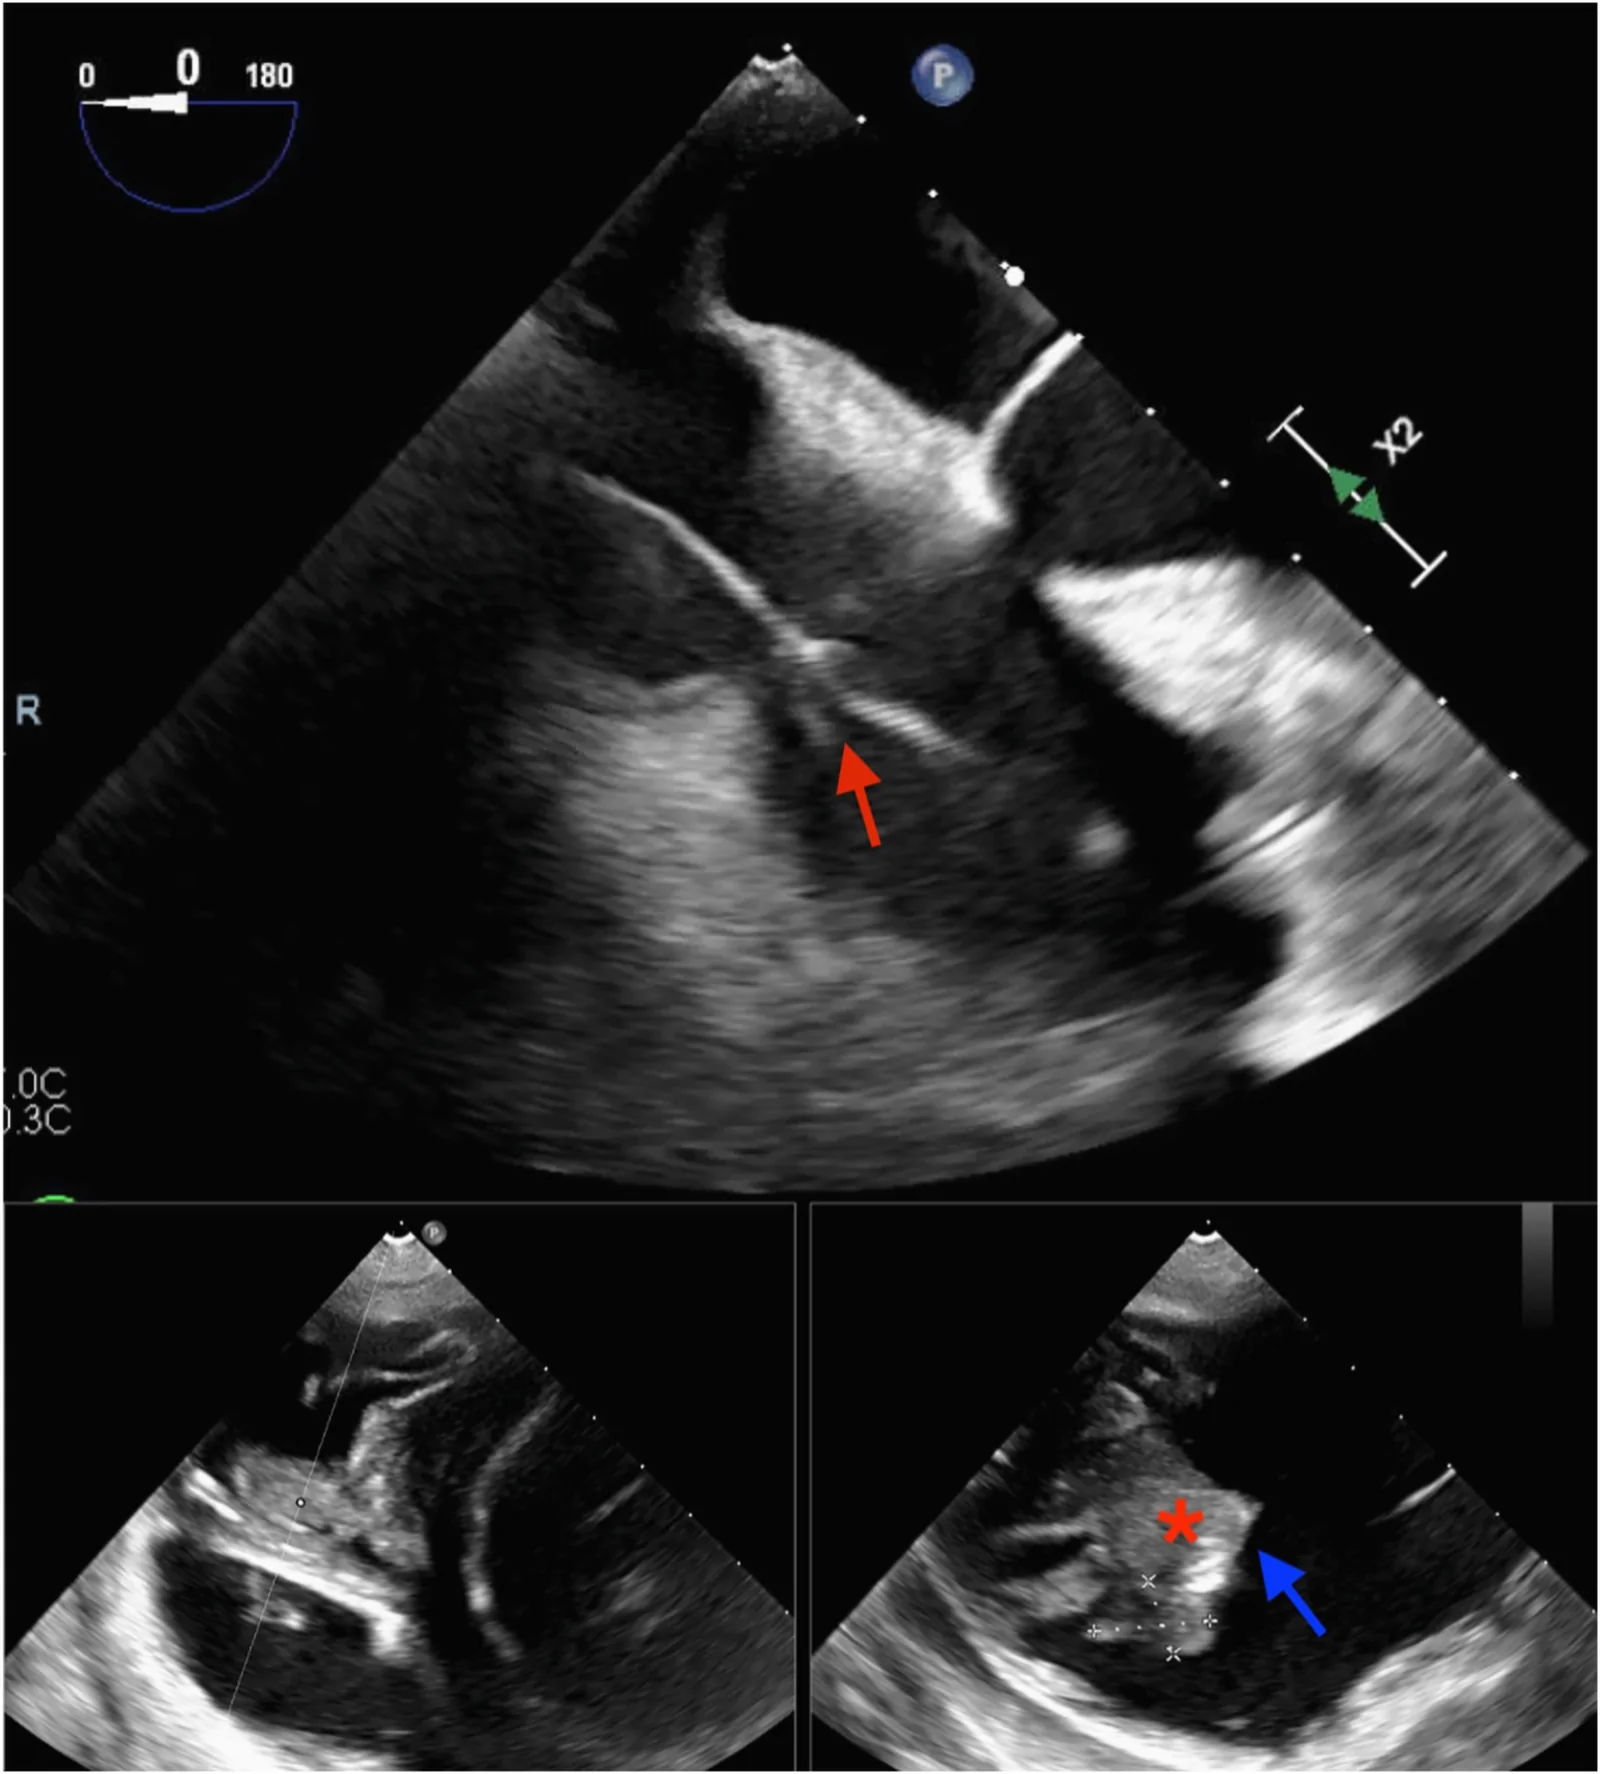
Transesophageal echocardiogram showing infective vegetations on both cardiac implantable electronic devices. (*Red arrow*) Infective vegetation on the right atrial portion of the ventricular lead. (*Red star*) Body of the leadless pacemakers. (*Blue arrow and markers*) Associated infective vegetation on the leadless pacemakers and subvalvular apparatus.
